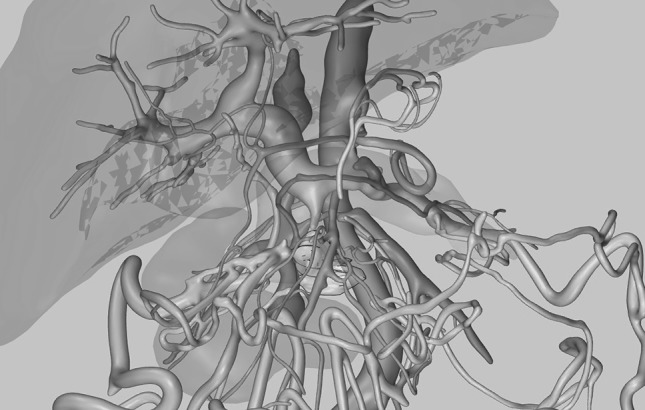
3D reconstruction of preoperative CT-scan for patient 1
